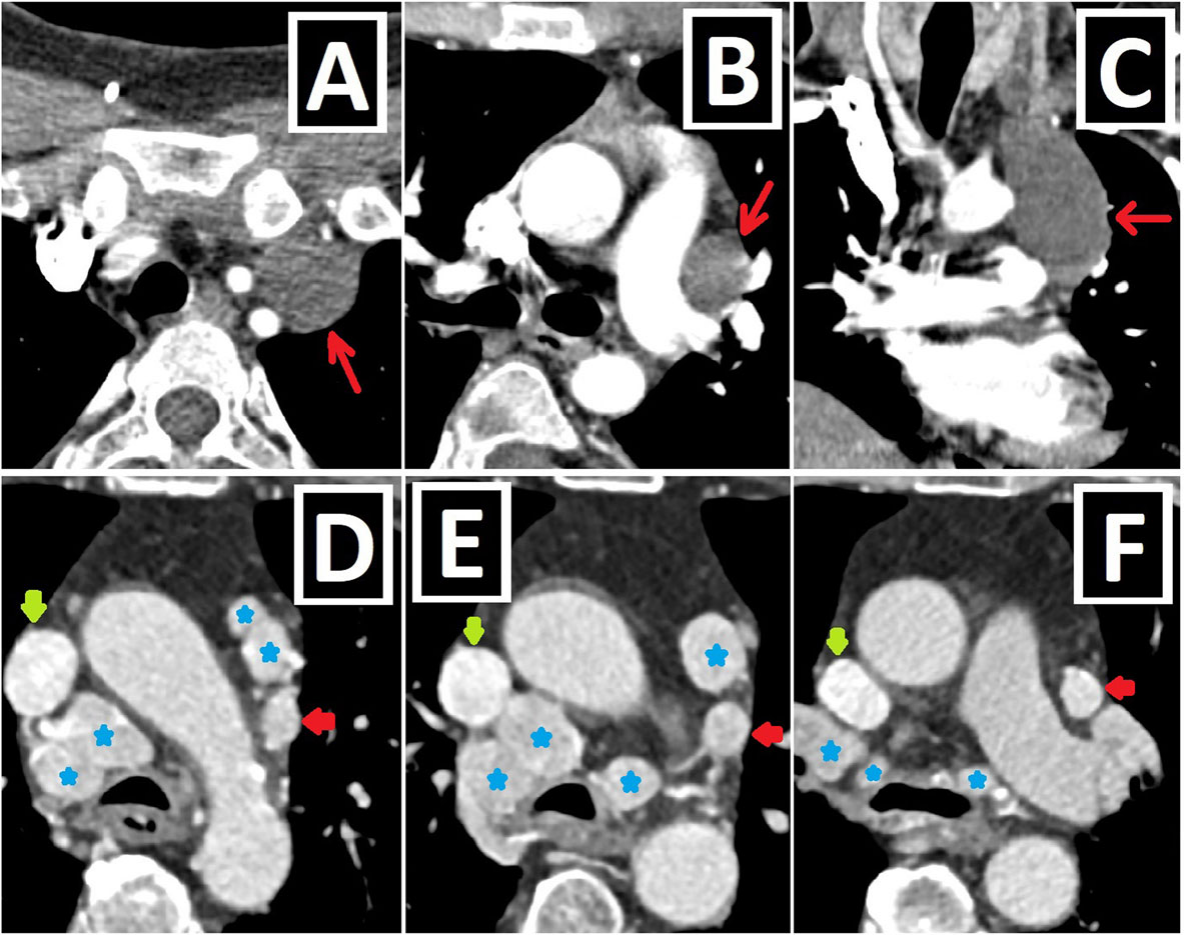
Masses mimicking PLSVC. a–c Axial (a, b) and coronal-oblique reformated (c) CT images depict a neurofibroma (red arrows) arising from the left phrenic nerve, which has a parallel course with the pericardiophrenic vein, in a patient with neurofibromatosis type 1. If the beginning/end of the mass and relationship with vascular structures are not carefully evaluated, it can be confused with PLSVC due to its location. d–f Axial CT images depict multiple mediastinal hypervascular lymphadenopathies (blue stars) in the patient with renal cell carcinoma. Hypervascular lymphadenopathies in the left half of the mediastinum (red arrows) may mimic PLSVC. Green arrows show RSVC
